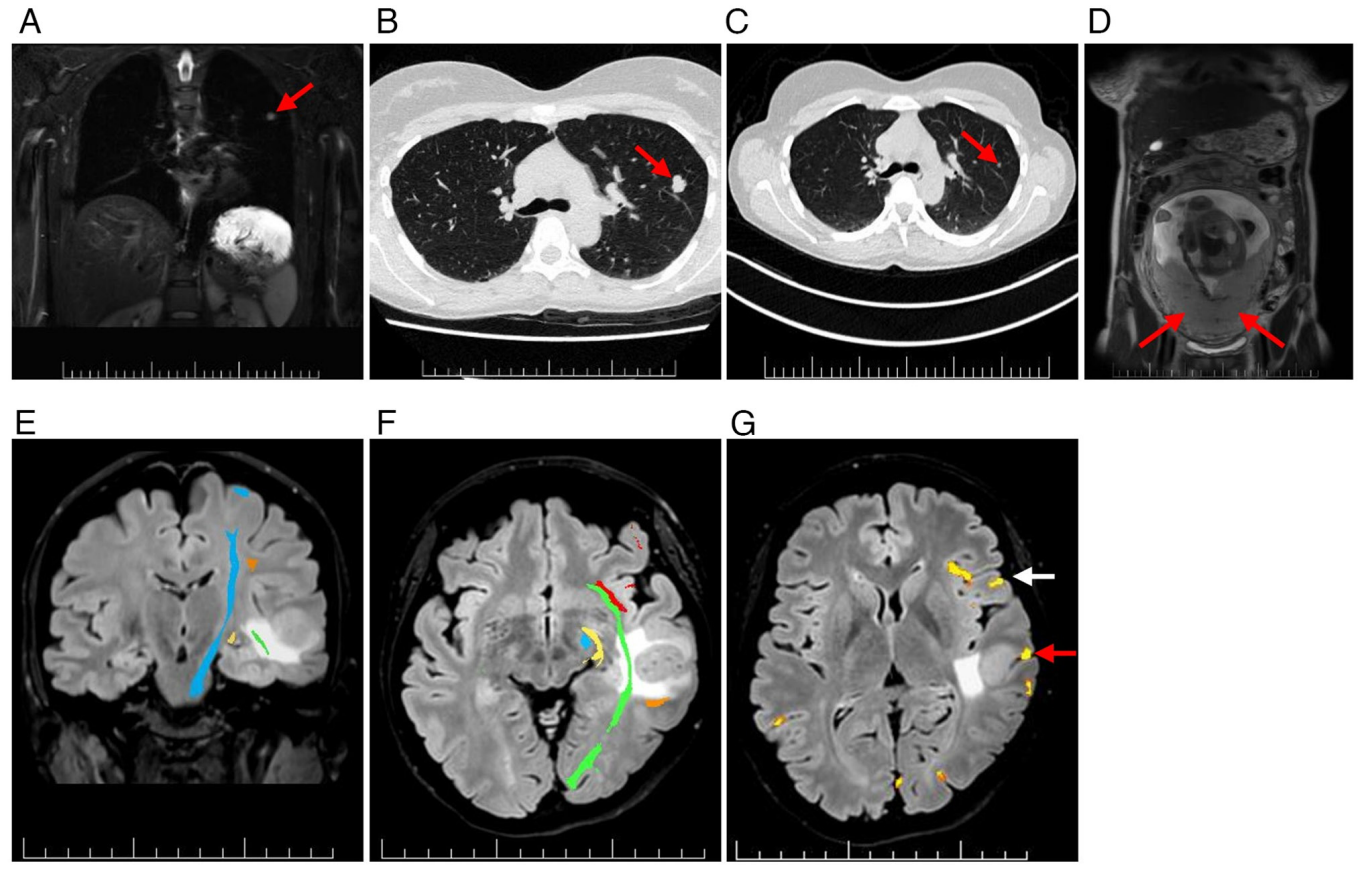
WB-MRI, HRCT pulmonary scans and pre-surgical brain mapping in relation to the left temporal metastasis. (A) WB-MRI confirming the pulmonary nodule in the left upper lobe (red arrow). (B) HRCT scan showing a lobulated pulmonary nodule in the same region (red arrow; January 2024). (C) Pulmonary nodule visible on CT in January 2023 (red arrow). (D) Placenta with homogeneous appearance and no evidence of metastatic involvement (red arrows; coronal T2-weighted image). (E) Diffusion tensor imaging demonstrating major white matter tracts in proximity to the lesion: Corticospinal tract (blue), inferior fronto-occipital fasciculus (green), arcuate fasciculus (orange) and optic radiation (yellow). (F) Tractography showing all major tracts, including the uncinate fasciculus (red). (G) Functional MRI demonstrating eloquent cortical areas relevant to speech (yellow); Wernicke's area (red arrow), Broca's area (white arrow). Scale bars, 10 mm. WB, whole-body; HRCT, high-resolution computed tomography.
